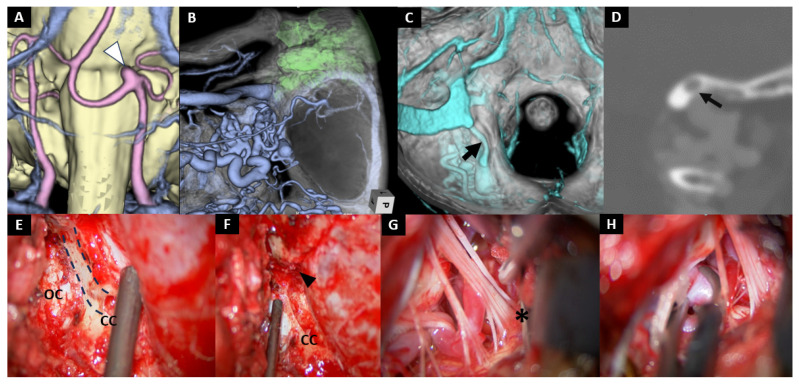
Case 9: Left transcondylar fossa approach for an VA-PICA aneurysm. (A) Volume rendering was performed on T1-weighted thin-slice images including the brainstem, and the dataset was fused with magnetic resonance angiography (MRA) on a workstation to delineate the location of the aneurysm. The aneurysm (white arrowhead) is located at the left pontomedullary junction. (B) 3D-reconstructed CT angiogram shows preoperative assessment of the anatomical relationship among the mastoid air cells, digastric groove, and sigmoid sinus. (C) Reconstructed 3D-CT angiogram identifies the course of the condylar emissary vein (arrow). (D) Coronal section of the CT angiogram shows that a well-developed condylar emissary vein makes outer cortical bone thin (arrow). (E–H) Intraoperative photographs. (E) The outer wall of the condylar canal (dotted line) is removed with a diamond burr. (F) The condylar canal is opened by coagulating and collapsing the condylar emissary vein up to the posterior margin of the sigmoid sinus (arrowhead). (G) Dissection of the arachnoid membrane around the root origin of the CN IX (*) allows mobilization of the cerebellum. (H) The cerebellum is gently elevated with a spatula to obtain the operative corridor, followed by aneurysm clipping. OC: occipital condyle; CC: condylar canal; SS: sigmoid sinus.
